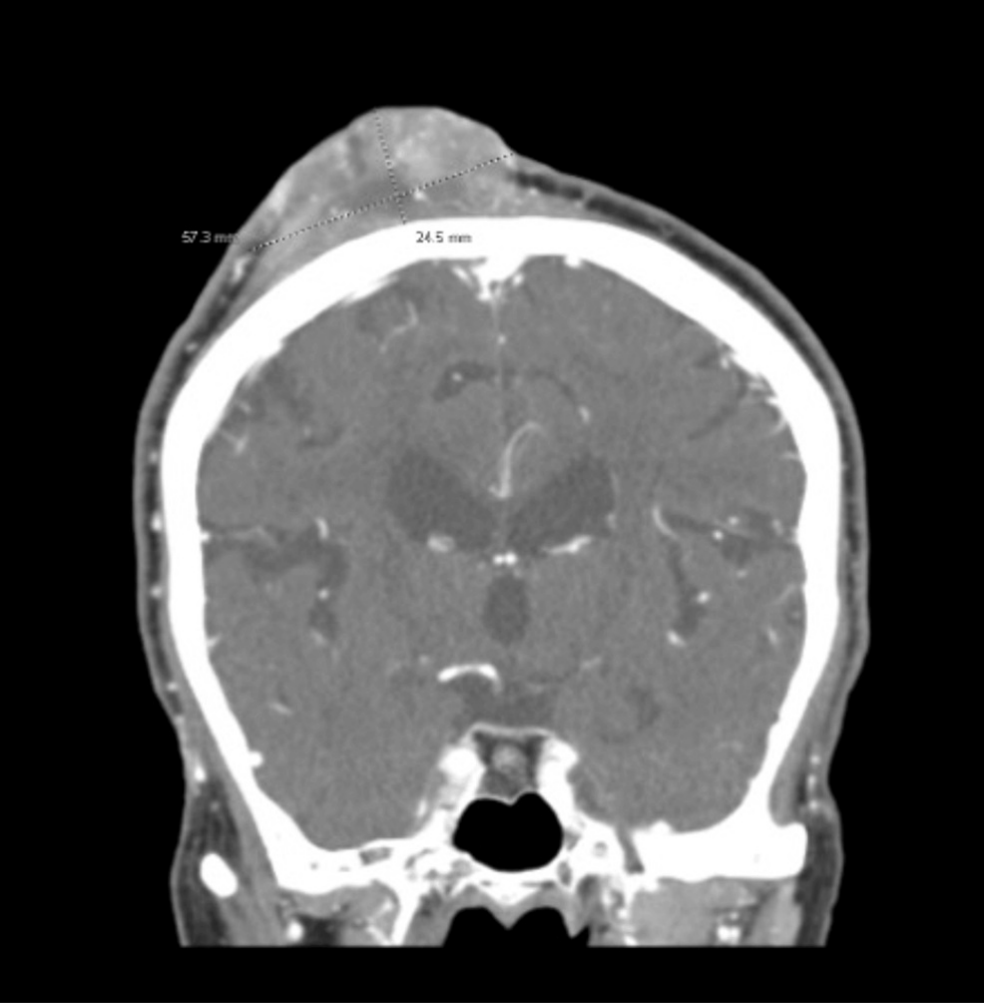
CT of the head with contrast showing mass overlying the right parietal scalp.
